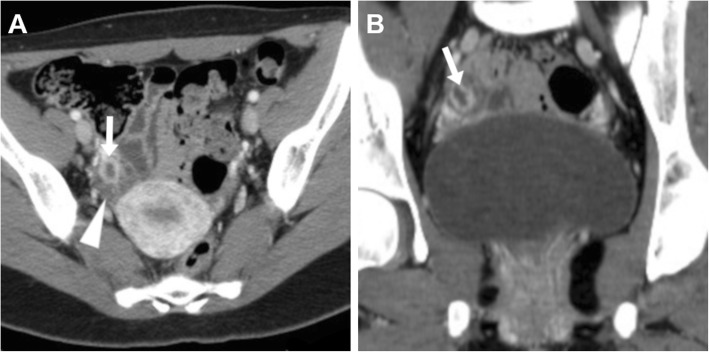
Typical appearance of an uncomplicated corpus luteum in the second half (luteal phase) of the menstrual cycle on axial (a) and coronal (b) CT images: the normal-sized right ovary (arrowhead) contains a 1.5-cm cystic structure demarcated by a crenulated, strongly enhancing peripheral rim (arrow)
